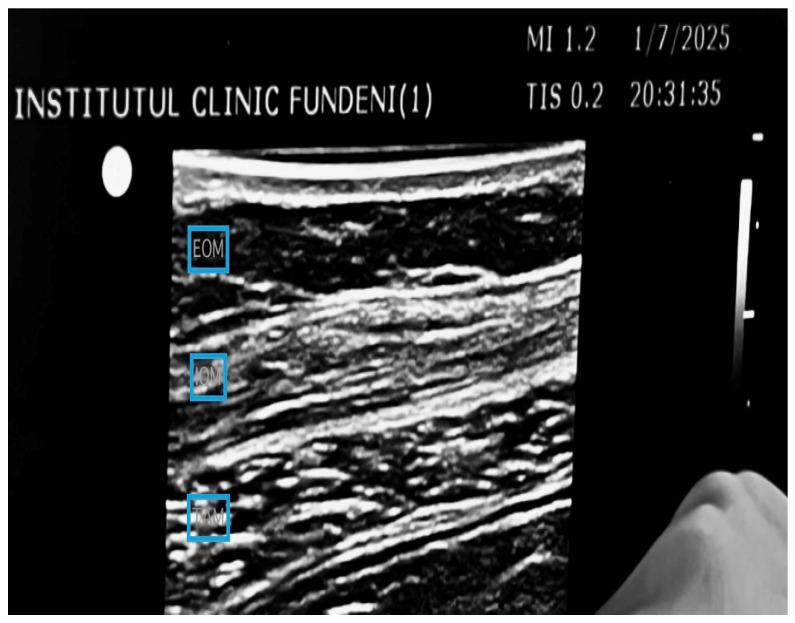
US TAP block approach: in the TAP group, the linear probe was placed in the transverse plane at the midaxillary line between the lower costal margin and iliac crest. When the EOM, IOM, and TAM were observed, a 20-gauge, 100 mm needle (Stimuplex^®^ Ultra 360^®^; B.Braun, Mihela Olita, Fundeni Clinical Institute, Bucharest, Romania, Affiniti 70, Philips Ultrasound from Fundeni Clinical Institute, Bucharest, Romania) was advanced using an in-plane technique in an anteromedial to posterolateral direction toward the TAP (the fascial plane between the IOM and TAM) (from the authors’ collection).
